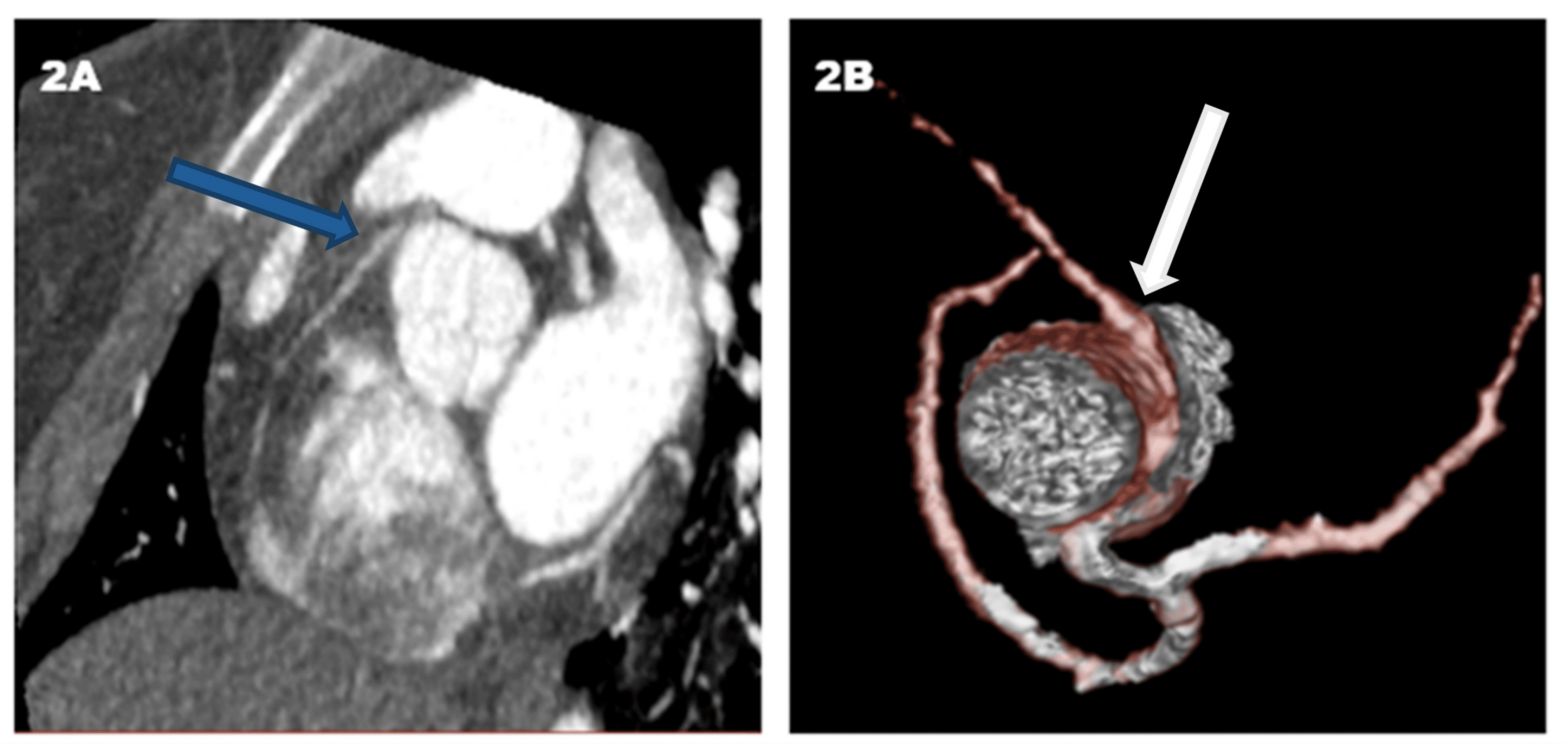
Case 2 Imaging (A) Cardiac computed tomography angiography (CTA) cross-view showing right coronary artery (RCA) coursing between the aorta and pulmonary trunk (blue arrow). (B) Three-dimensional reconstruction image showing the smaller diameter RCA when compared with the left coronary artery (LCA) due to compression of the RCA between the aorta and pulmonary trunk (white arrow). Image credit for B: Dr. Malek Othman.
